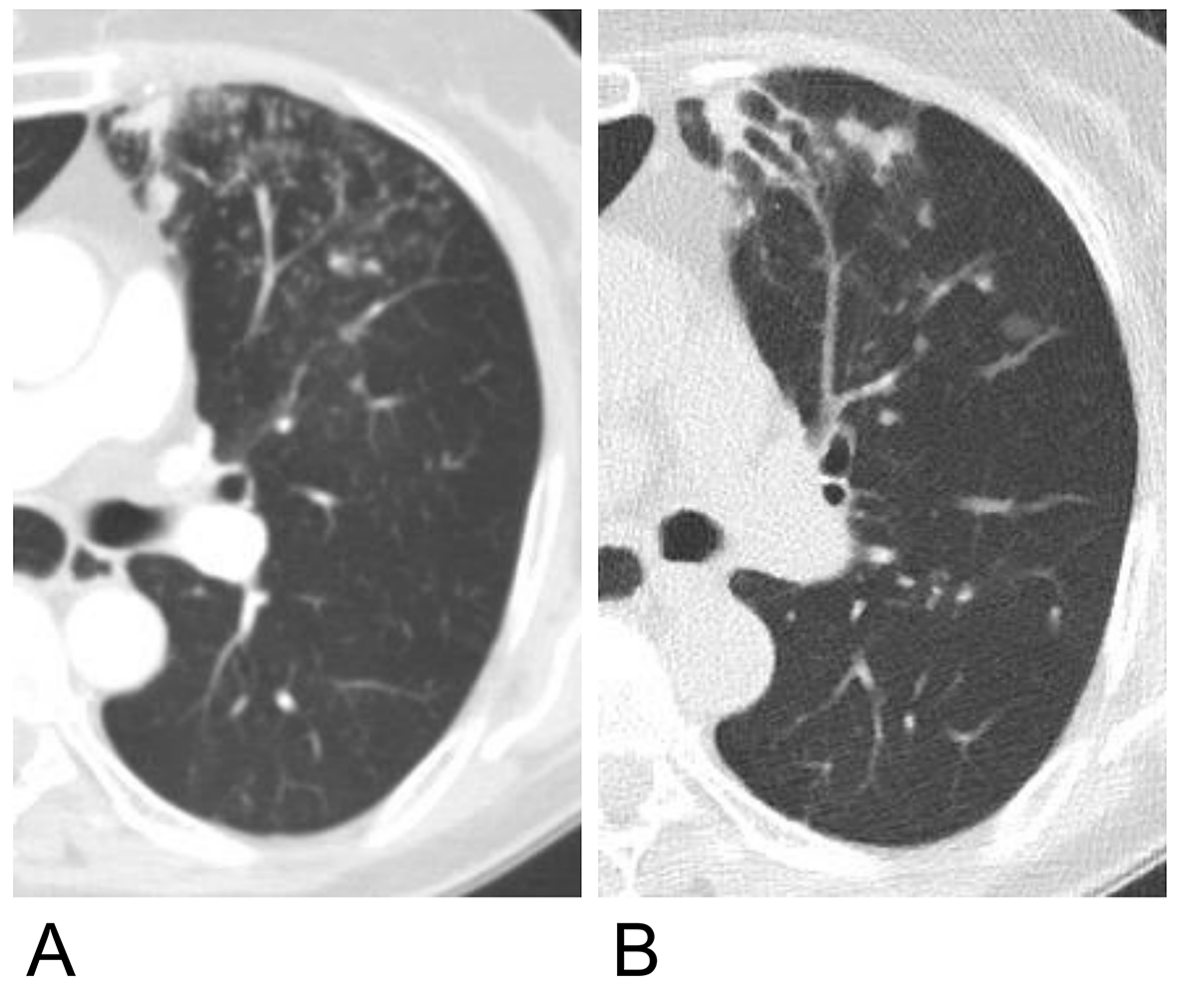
Chest CT images of a female patient. (A) The initial CT image showed no bronchiectasis. Centrilobular and small nodules were observed in the anterior segment of the left upper lobe. (B) Bronchiectasis became evident after 5 years and centrilobular nodules were still observed but to a lesser extent. CT, computed tomography
